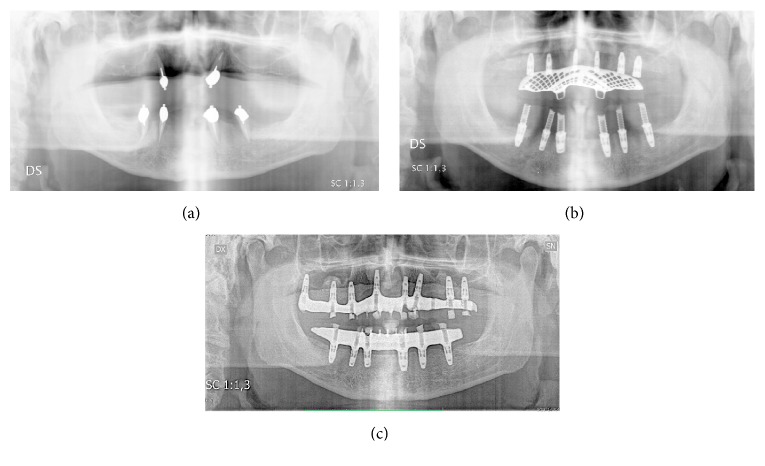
Orthopantomography of the patient at first visit (a), 18 weeks (b), and 96 weeks (c).
